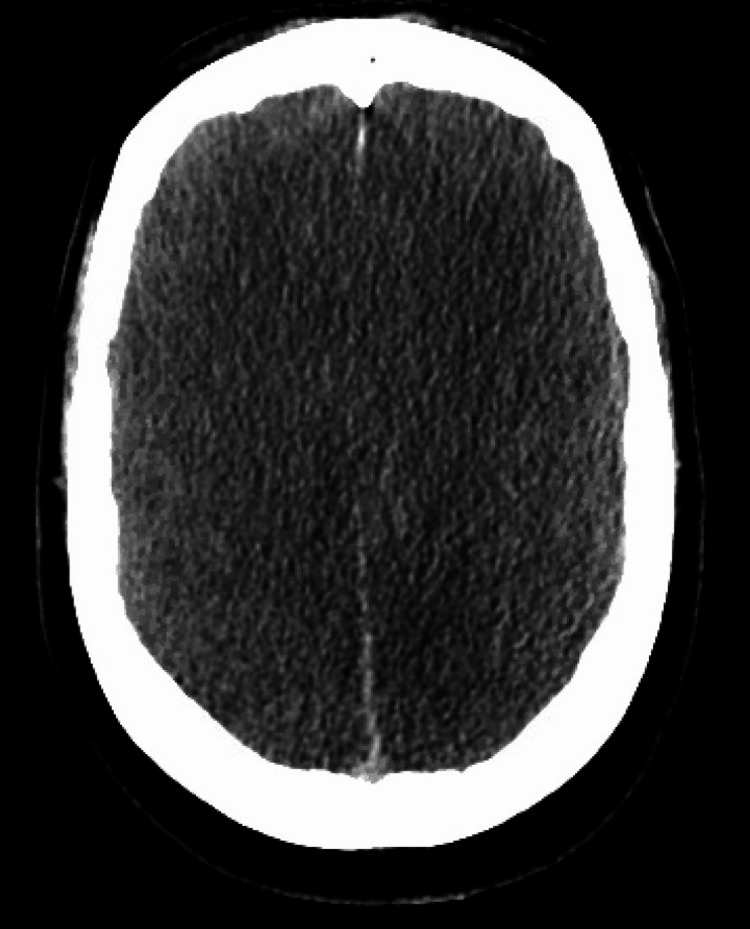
Axial CT scan of the head shows complete loss of gray-white matter differentiation and complete obliteration of brain sulci and cisterns suggesting diffuse anoxic brain injury CT, computerized tomography
